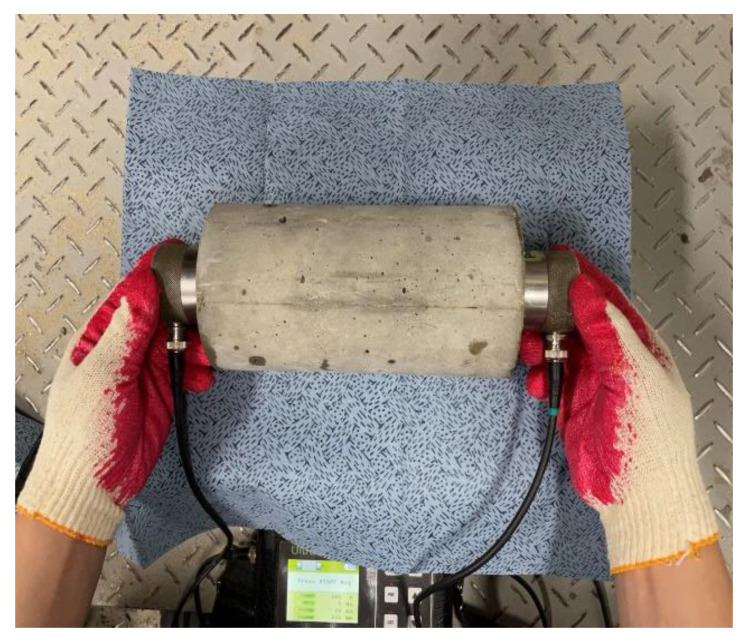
Ultrasonic pulse velocity test [22].
